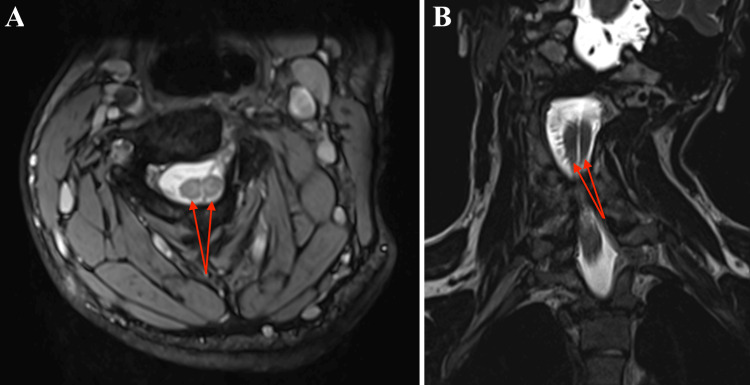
Cervical spine MRI MRI, magnetic resonance imaging (A) The axial plane of the MRI showing two hemicords within a single dural sac (red arrows). (B) The coronal plane of the MRI showing two hemicords (red arrows).
